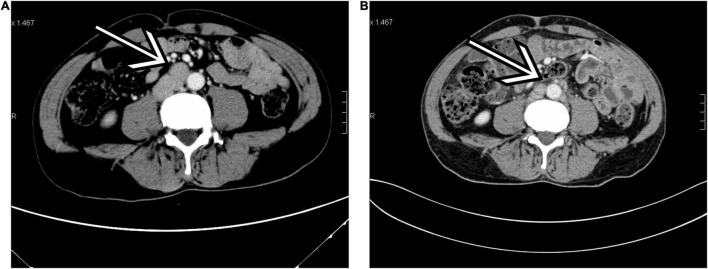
A 50-year-old man with Sertoli cell tumors (SCT; case 10). (A) Transverse contrast-enhanced CT (arterial phase) performed before orchiectomy showed enlarged para-aortic lymph nodes (arrow). (B) The same level of contrast-enhanced CT (arterial phase) performed 40 months after orchiectomy found that the enlarged para-aortic lymph nodes had diminished (arrow). *p* < 0.01, p* < 0.001.
